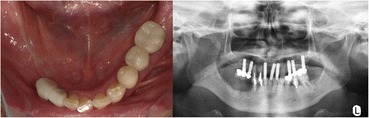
Postoperative intraoral finding and radiograph
